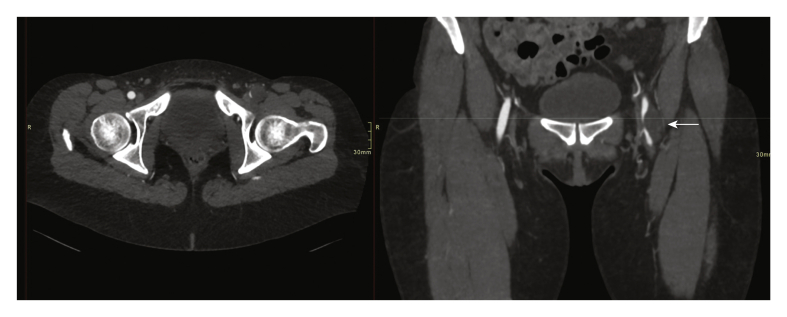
CT reconstructions in the axial (left) and coronal (right) plane at the level of the common femoral artery. The coronal image shows the slit like lumen of the common femoral vein in relation to the hypodense cyst (arrow).
